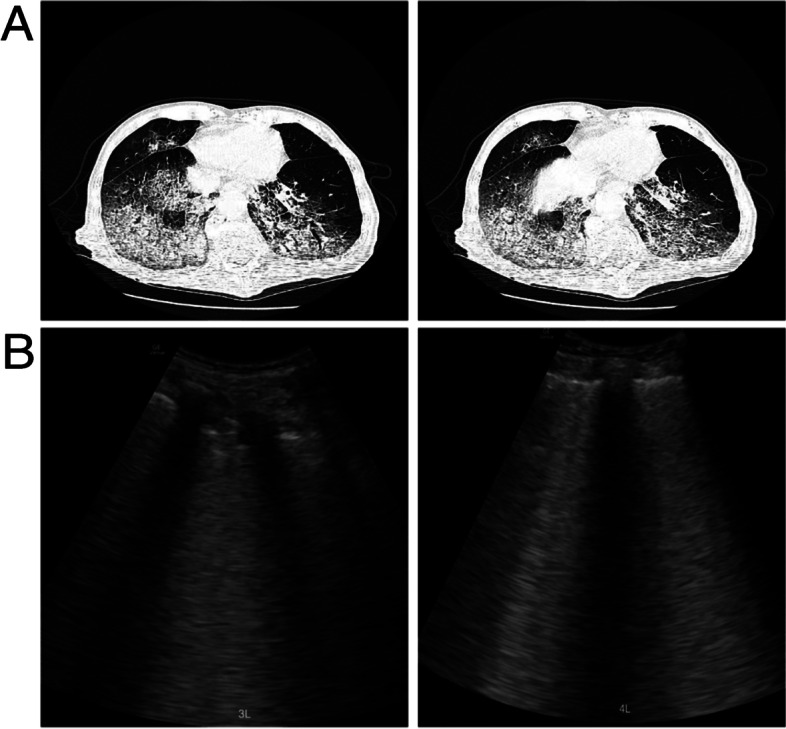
CT and LUS examination of a 78-year-old male COVID patient. A CT images showing the changes in the subpleural-peripheral in the dorsal zones of both lungs. B LUS shows multiple coalescent B-lines visible during the prone ventilation
